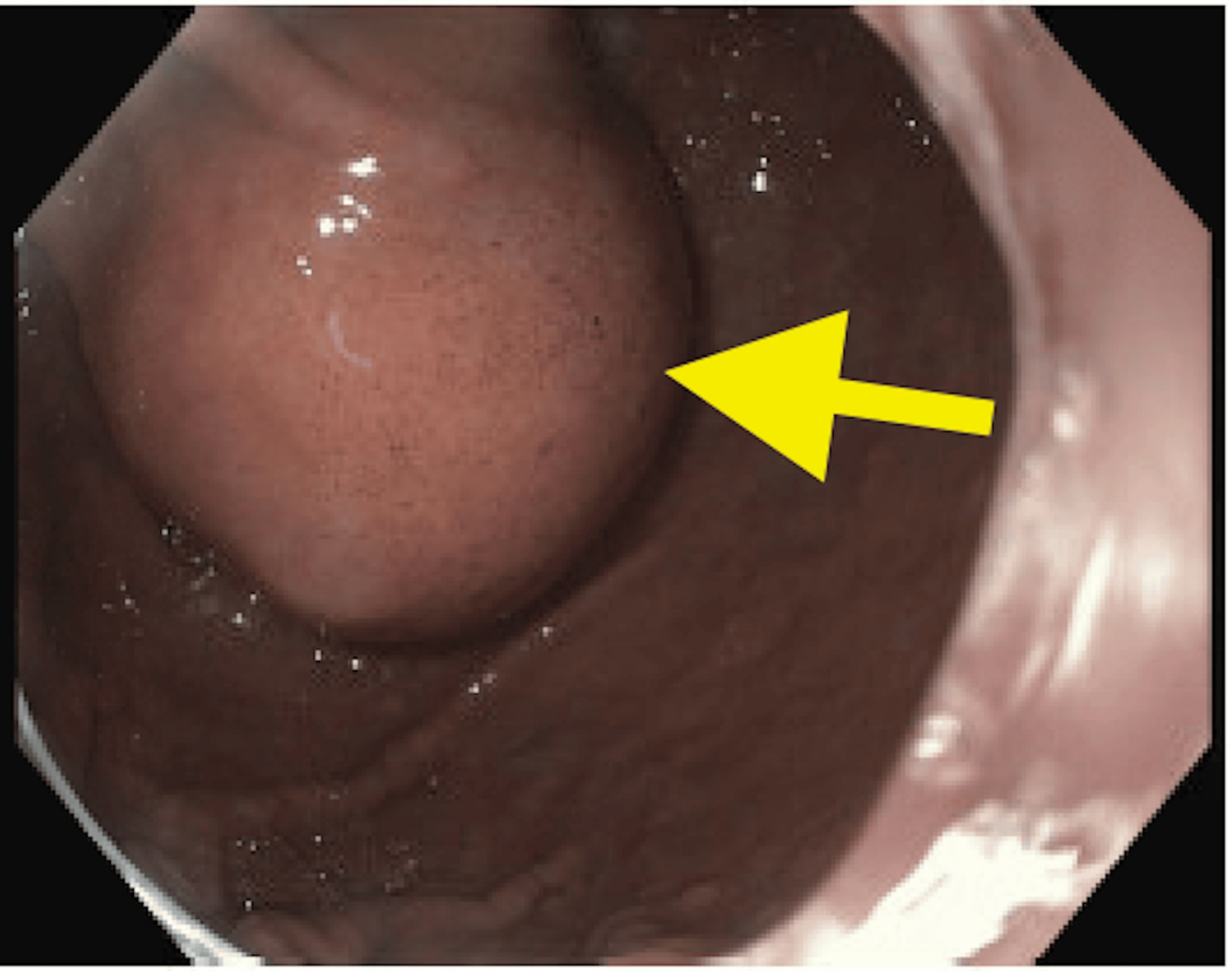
Endoscopic ultrasound showing antral mass (arrow)
